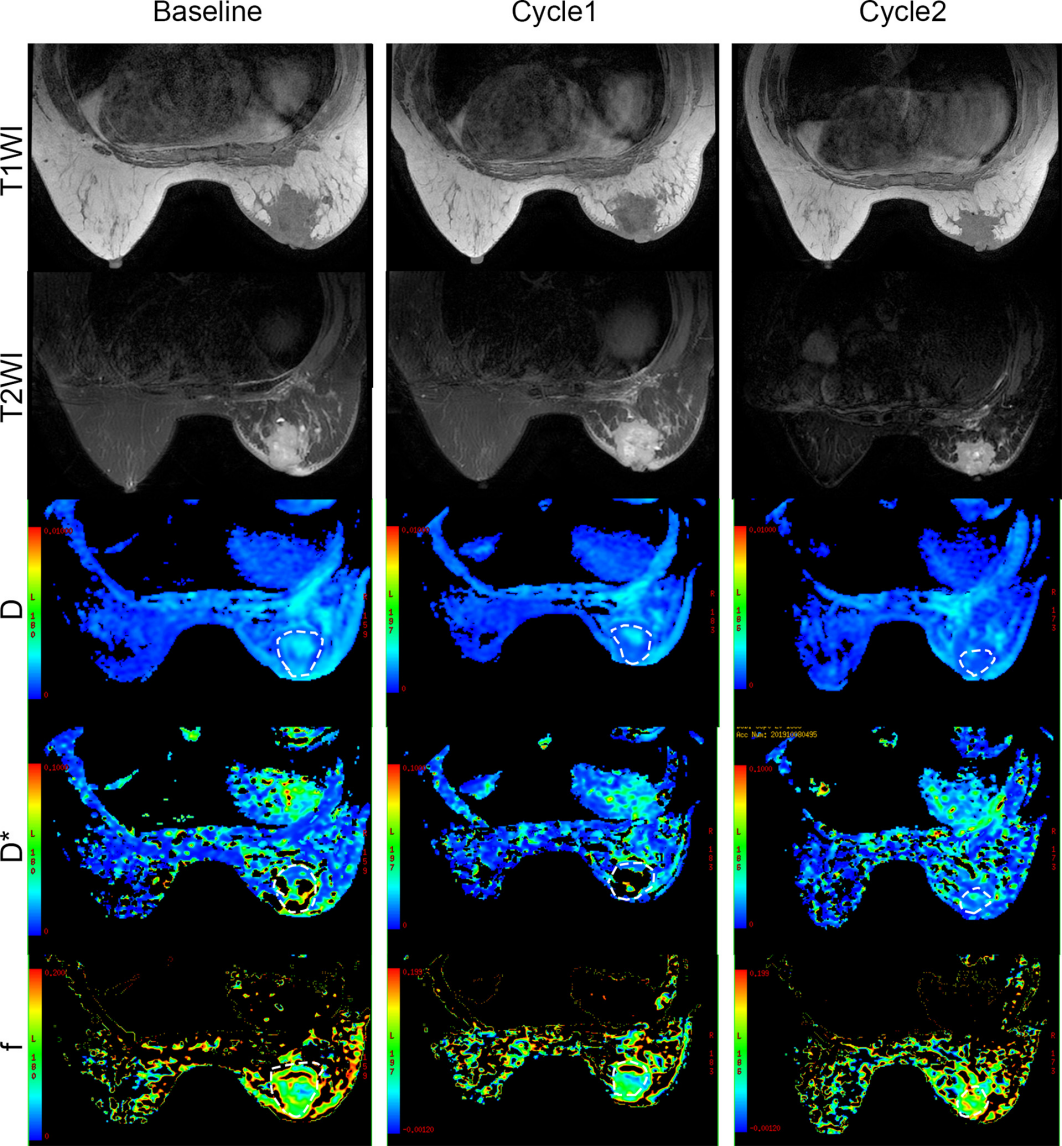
Conventional MRI (T1- and T2-weighted images) and functional maps in breast cancer patients with two cycles of immunochemotherapy. Dashed outlines denote tumor areas. Color ranging from blue to red represents values ranging from low to high.
